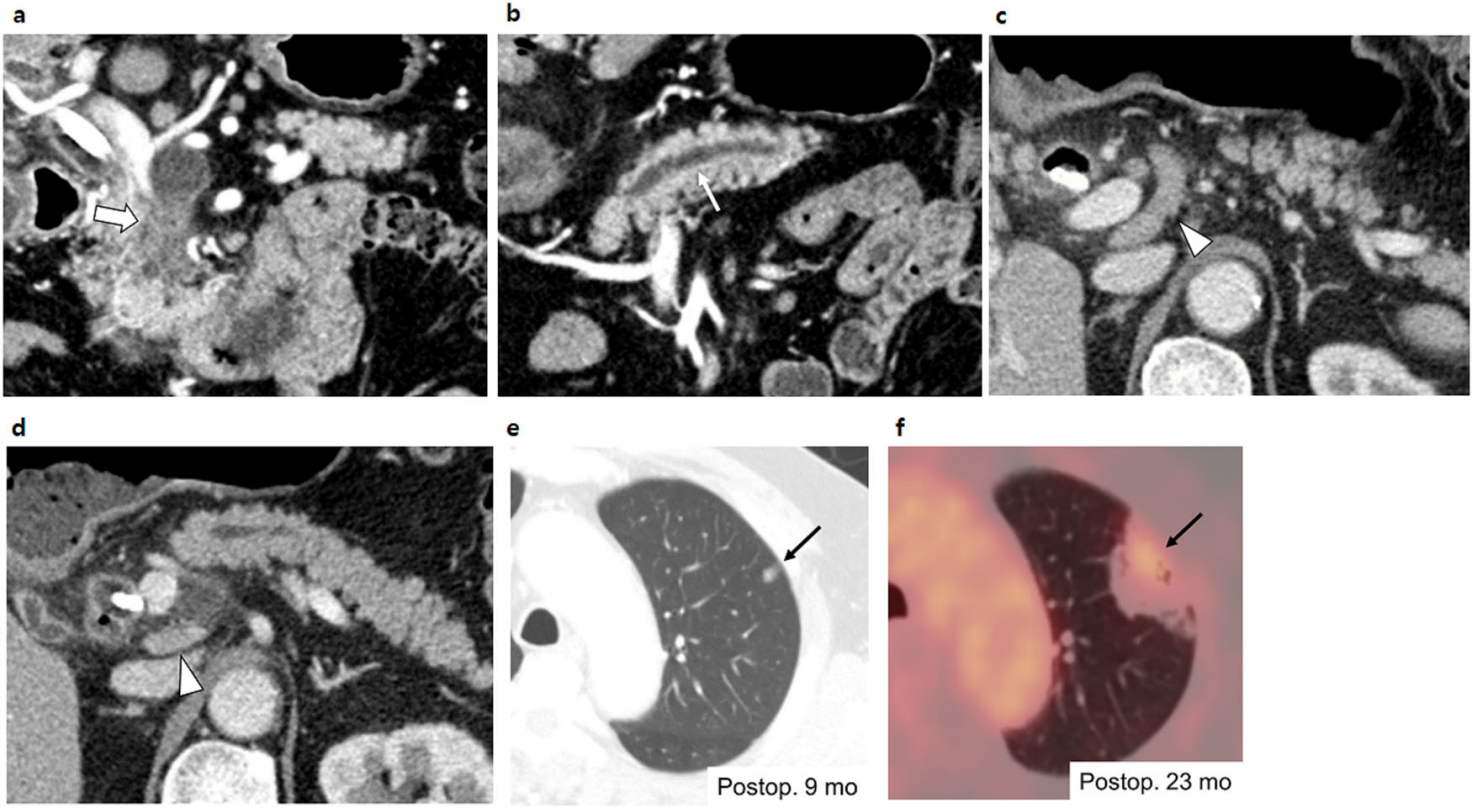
A representative case of a 57-year-old male patient who underwent surgery for IPMN. a On the preoperative CT, there was an approximately 5 cm pleomorphic cystic lesion with an EMN (thick arrow) in the uncinate process of the pancreas, which was thought to be IPMN with high-risk stigmata. b The MPD (white arrow) was mildly dilated to 5 mm, and c, d several enlarged lymph nodes (arrowheads) were observed around the lesion. After surgical resection, the lesion was confirmed to be IPMN with an associated invasive carcinoma, and two metastatic lymph nodes were identified. e During postoperative follow-up, a small lung nodule (black arrow) developed 9 months after surgery, which was reported to be a possible metastasis. f This was confirmed to be a metastasis as it had increased in size and F-18 fluorodeoxyglucose uptake (black arrow) on follow-up imaging
